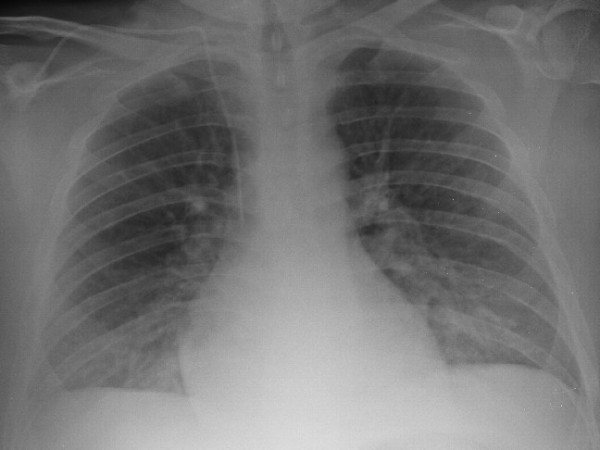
Chest radiograph showed significant improvement after treatment with systemic corticosteroids.
